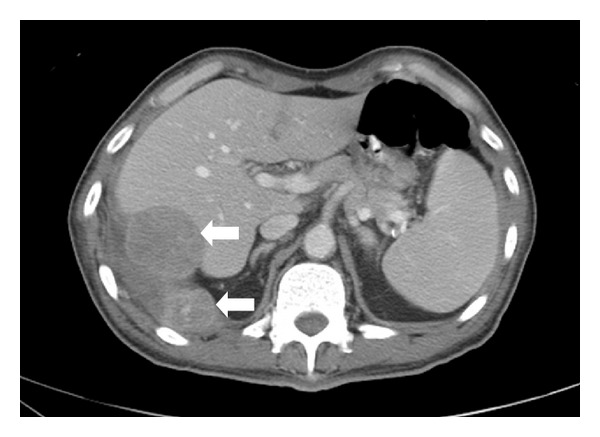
An axial chest, abdominal, and pelvic contrast-enhanced computed tomography (CT) scan showing bilateral pleural effusion, partial bibasilar atelectasis, and peripheral dense calcifications of the right lung. In addition, two pleural masses touching the right hemidiaphragm were identified and measured 3.2 × 2.1 cm and 4.3 × 4.8 cm (white arrows). The masses were suspicious for neoplastic lesions. No axillary or mediastinal lymphadenopathy was identified. Furthermore, no evidence of distant metastasis was identified.
